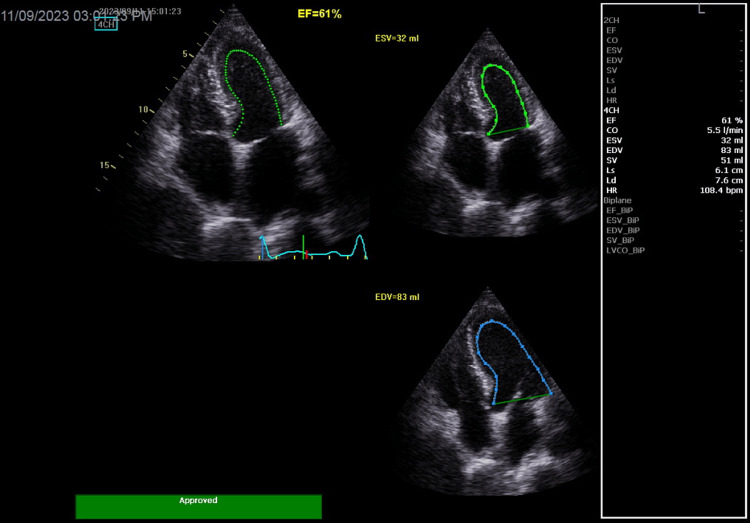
Baseline echocardiogram showing normal left ventricular ejection fraction at 61% with no wall motion abnormalities.
